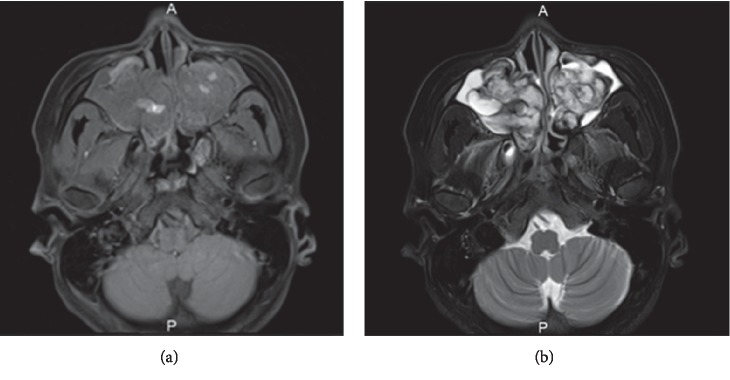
Magnetic resonance imaging (MRI). The mass as heterogeneous signal intensity arises from the bilateral maxillary sinus and extend to the nasal cavity on (a) T1-weighted image and (b) T2-weighted image.
